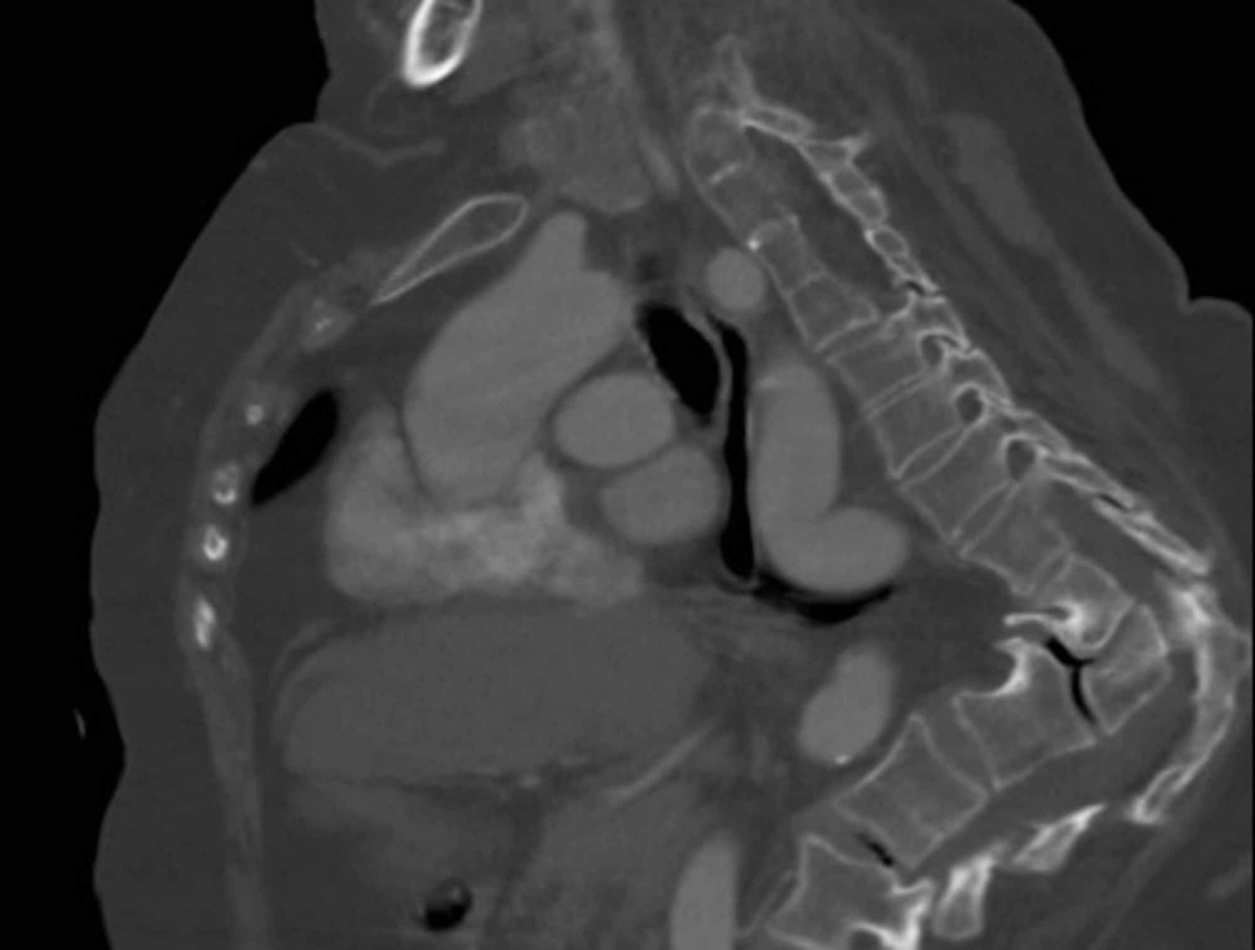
Chest computed tomography (CT), sagittal view, showing severe kyphoscoliosis of the spine.
